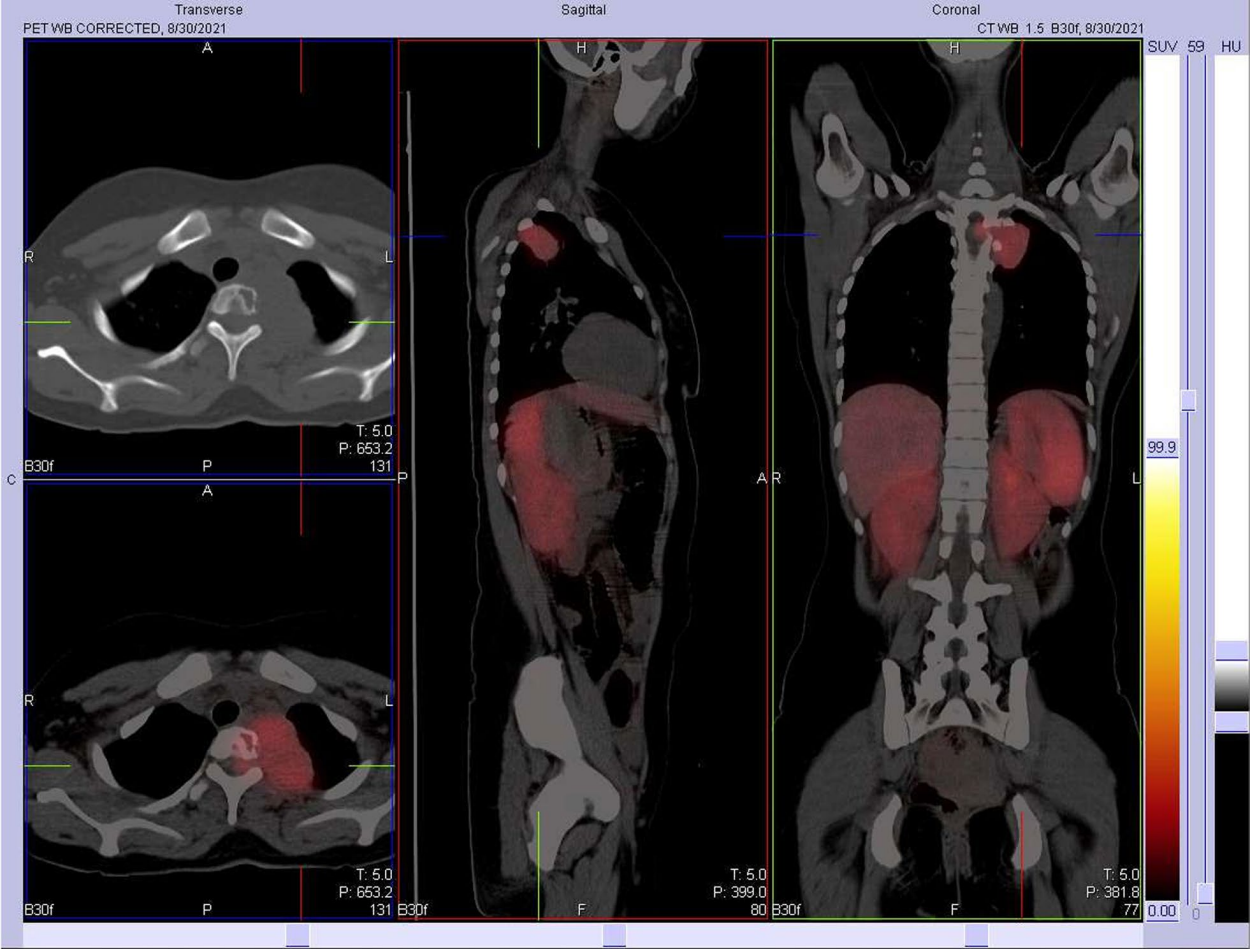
^68^Ga DOTATATE PET/CT Gallium Ga-68 DOTATATE scan showing receptor avid uptake in the left posterior mediastinal mass
